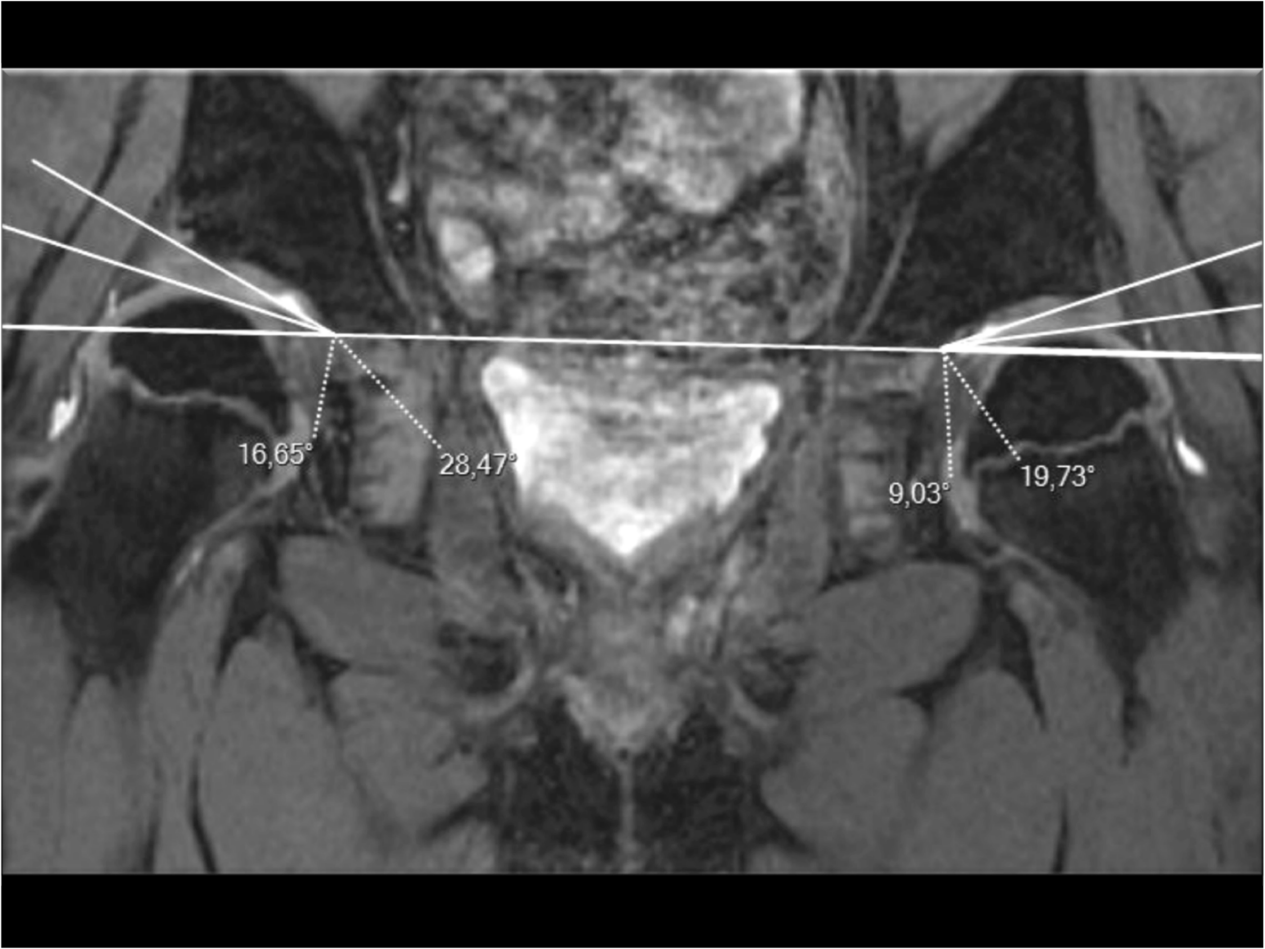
Measurements of Hilgenreiner’s angle on frontal MRI
